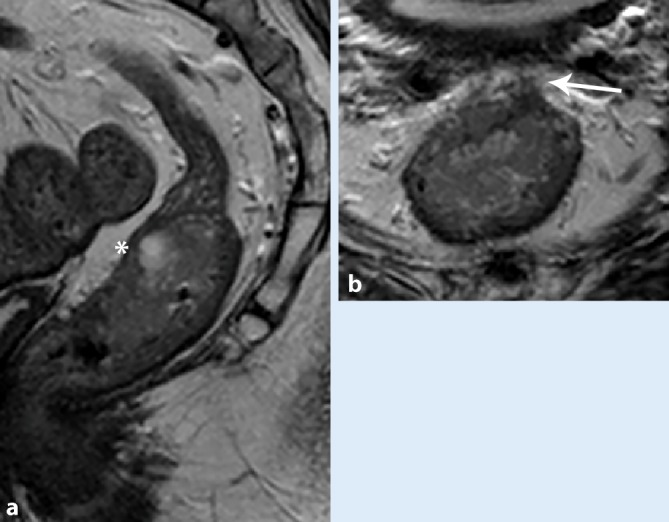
Sagittal and axial magnetic resonance images of a female patient with a T3ab tumor of the middle rectum (a; *white asterisk*) that penetrates the bowel wall on the anterior side (b; *black arrow*) but remains at a distance from the mesorectal fascia
